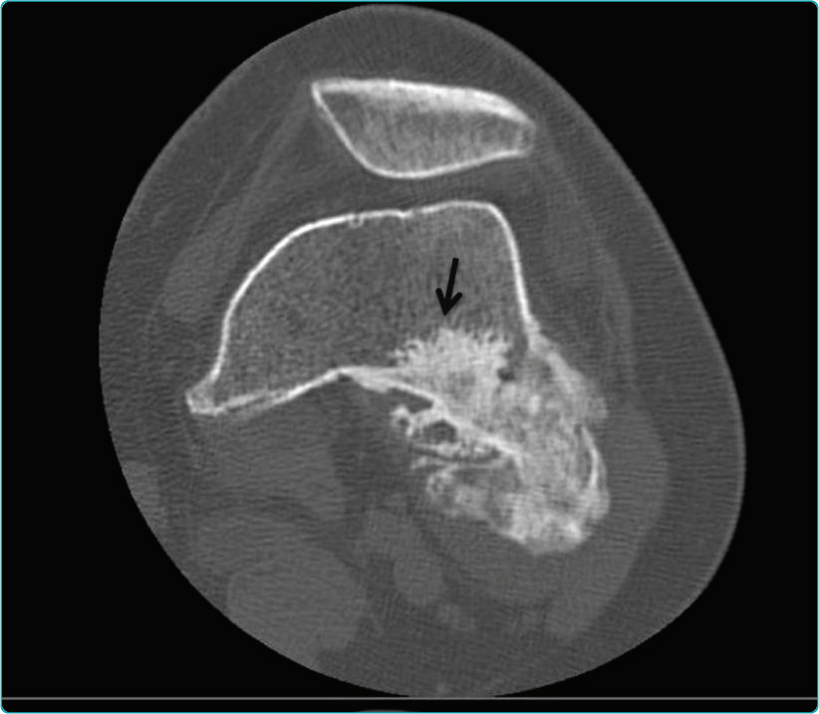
Case 2 - Computed tomography demonstrates an intact and continuous cortex along with communication of the medullary cavities. Limited invasion of the femoral medullar canal is also indicated (black arrow).
